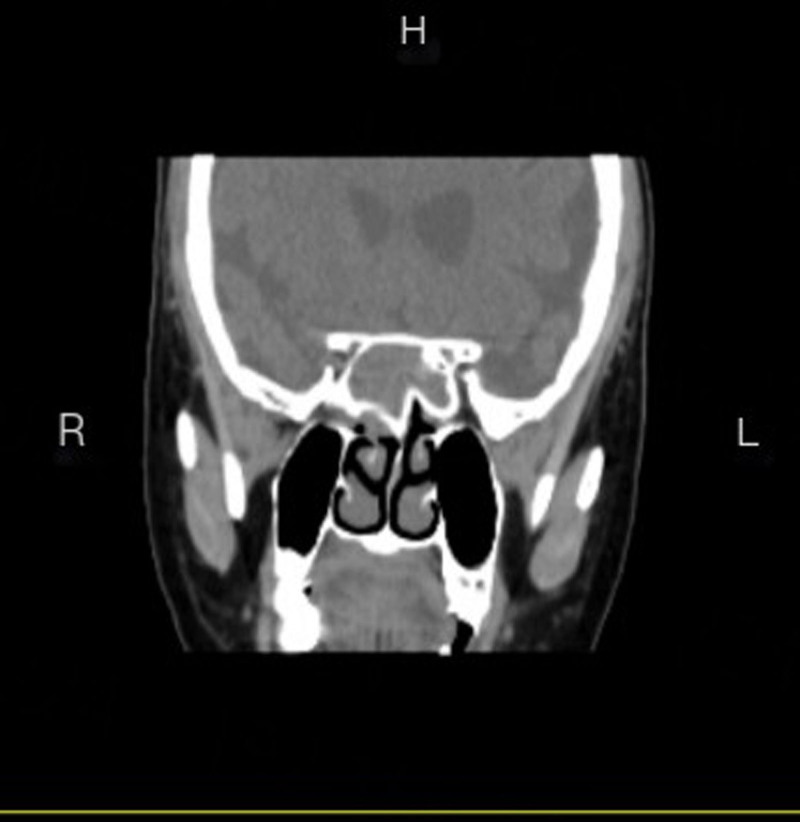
Patient, 70 years old, female, preoperative sinus CT reconstruction coronal. CT = computed tomography.
